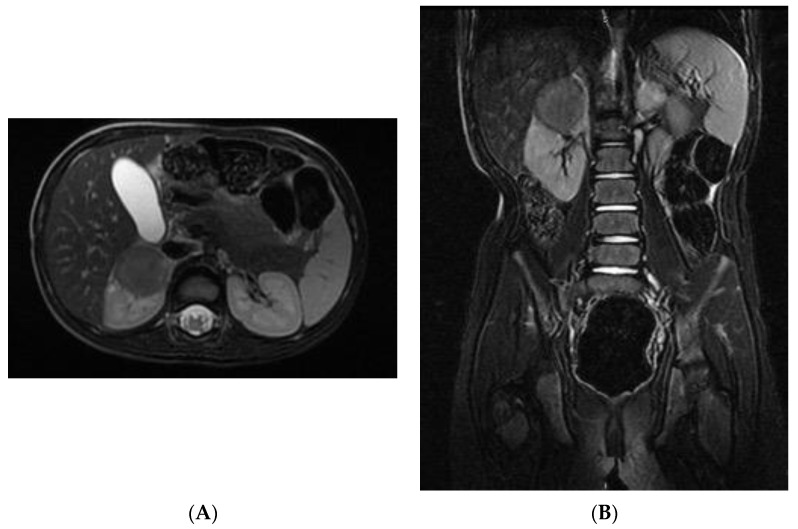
(A,B). Abdominal MRI: (A)—Axial view: the mass occupies the upper renal pole and appears partially exophytic. (B)—Coronal view: the mass entirely belongs to the upper renal pole and no lymph node localizations are detectable.
